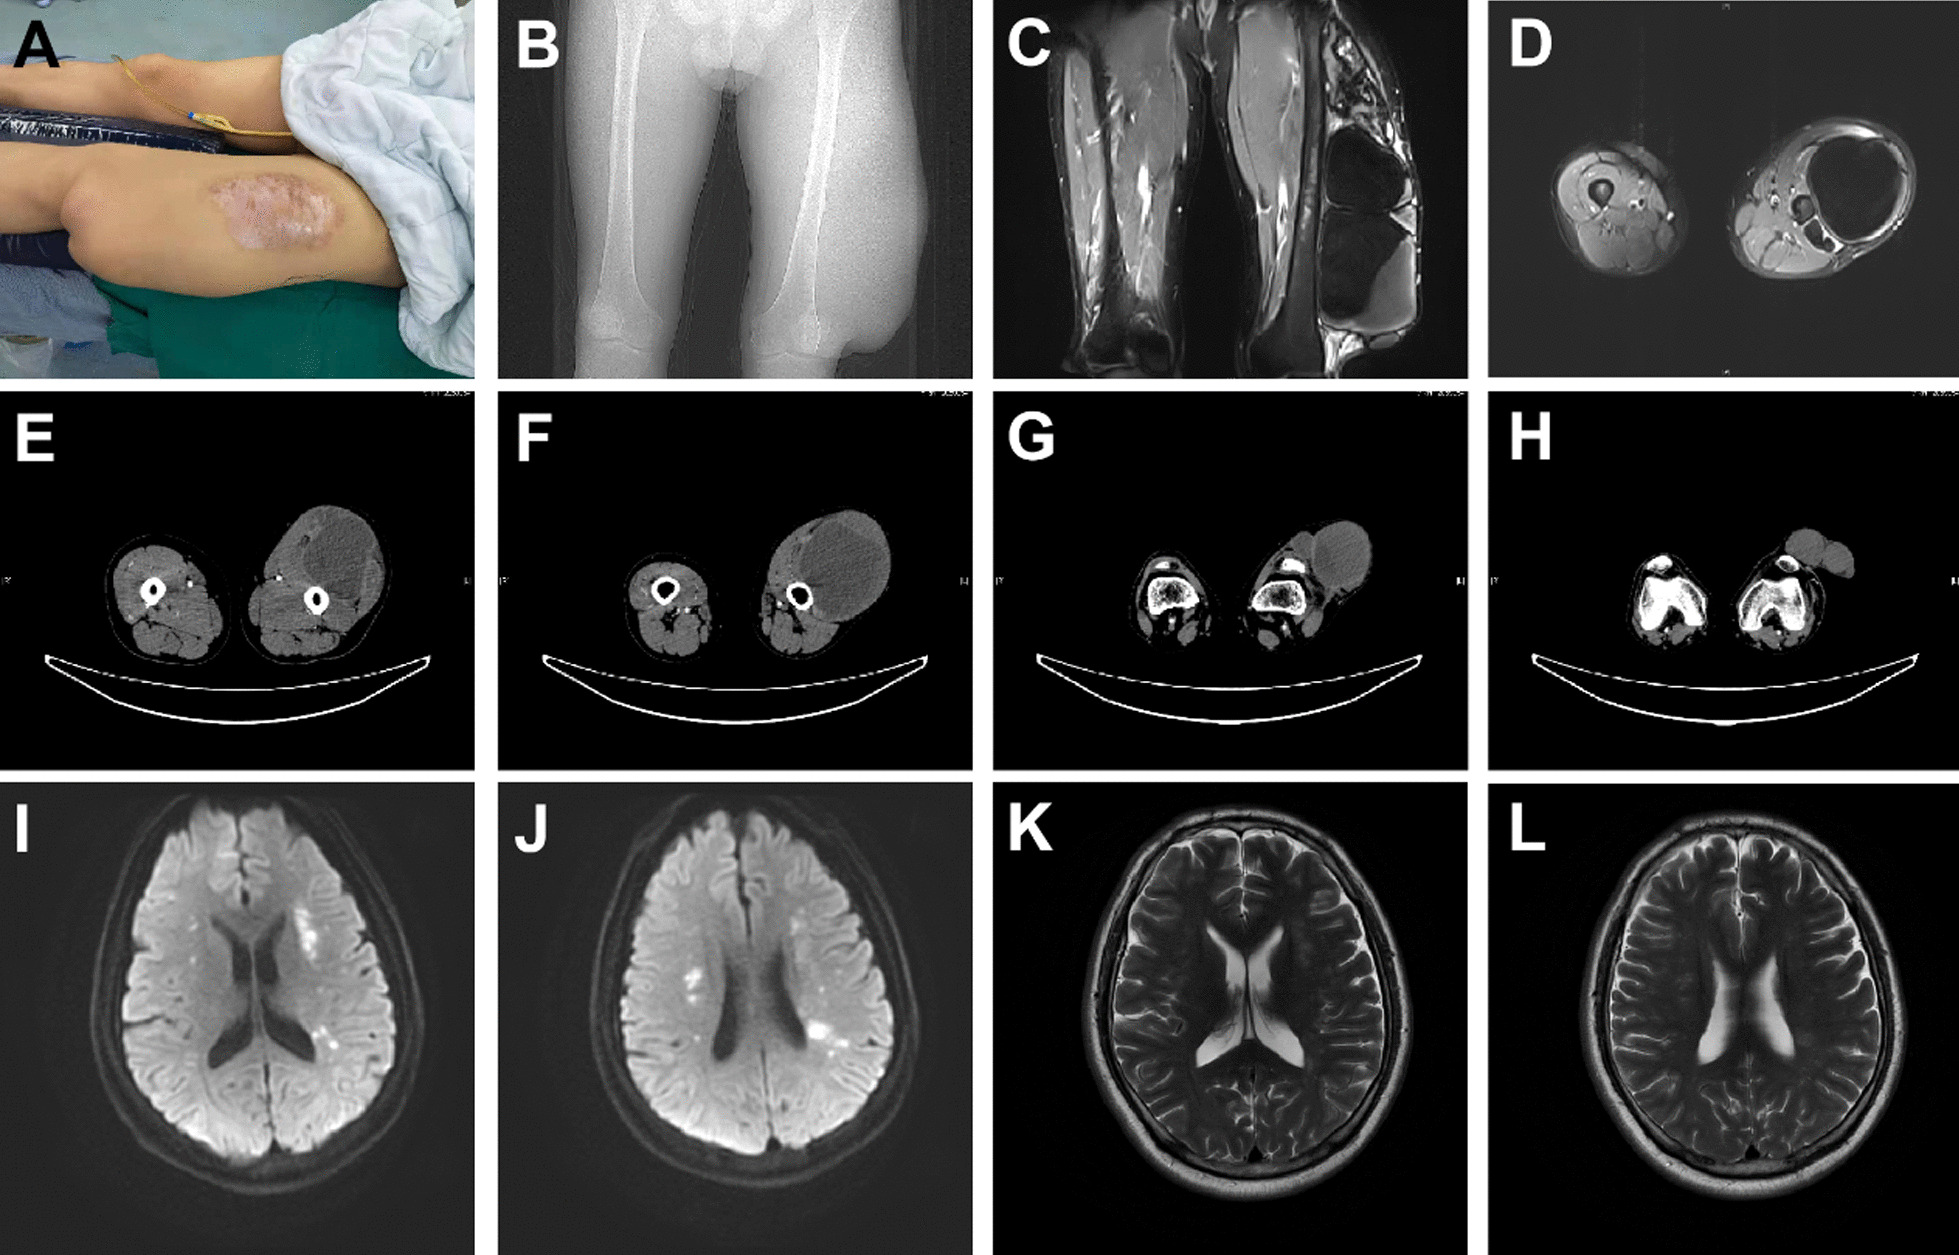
Preoperative photograph of left thigh showing a giant mass on the anterolateral region of left thigh (A). Anteroposterior radiograph of the left thigh showing a giant soft-tissue mass located at the left thigh without periosteal reaction of femur shaft (B). Coronal and axial Magnetic resonance imaging (MRI) of the left thigh showing the large pseudotumor masses on the anterolateral region of left thigh (C, D). Axial computerized tomography of leg showed intramuscular hematoma in left thigh (E–H). Diffusion weighted MRI and T2 weighted MRI of the brain showed there were multiple acute cerebral infarctions in bilateral lateral ventricles (internal border zone) and multiple ischemic foci in the white matter areas and basal ganglia of the bilateral cerebral hemispheres (I–L)
